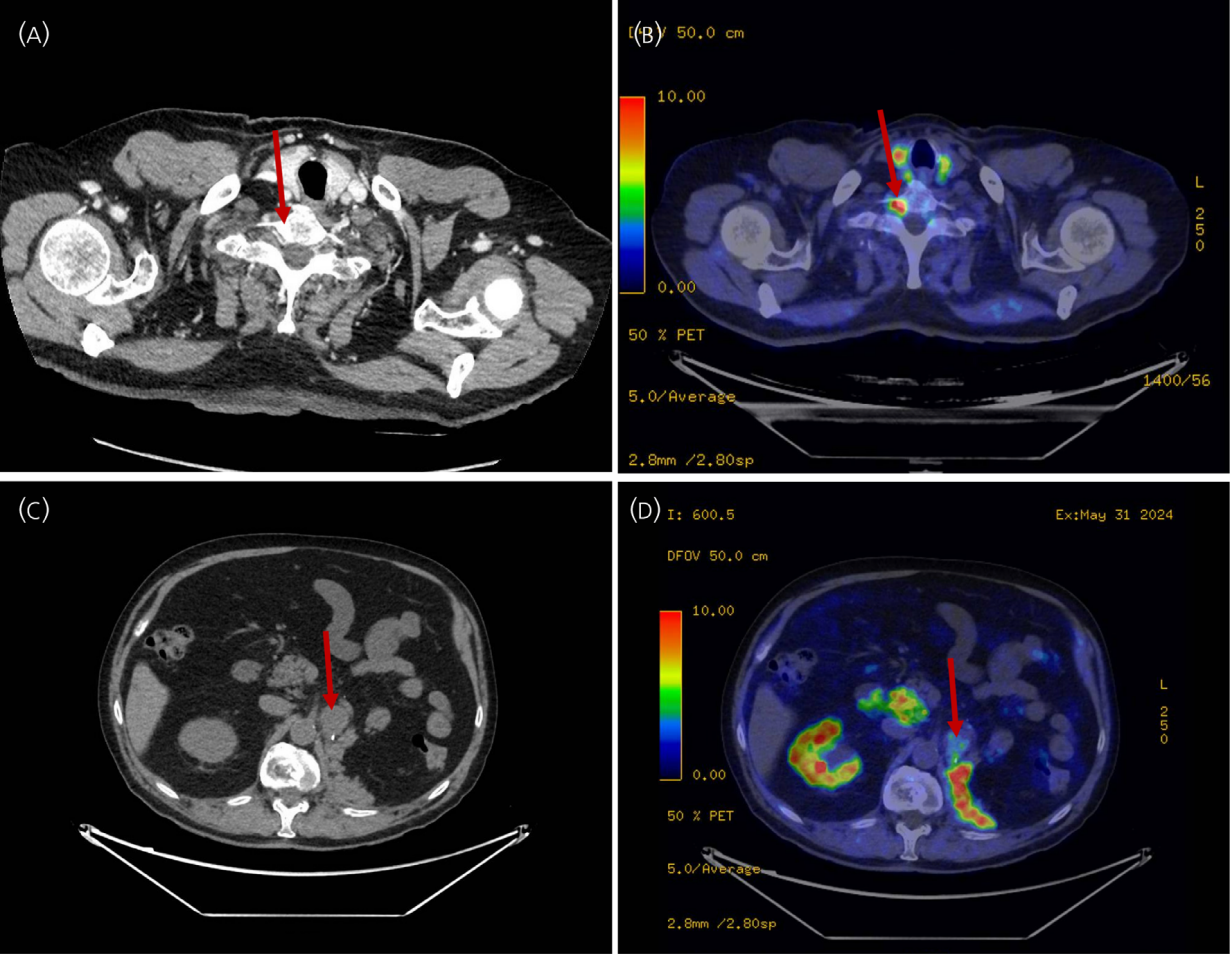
Discordant results in Patient 28 (A) CT of thorax with no sign of a C7 metastasis (arrow). (B) The C7 metastasis detectable with HED (arrow). Imaging results for *SDHC* patient (C) Local recurrence visible with CT (arrow). (D) Limited uptake of the HED‐PET/CT tracer in the recurrence.
